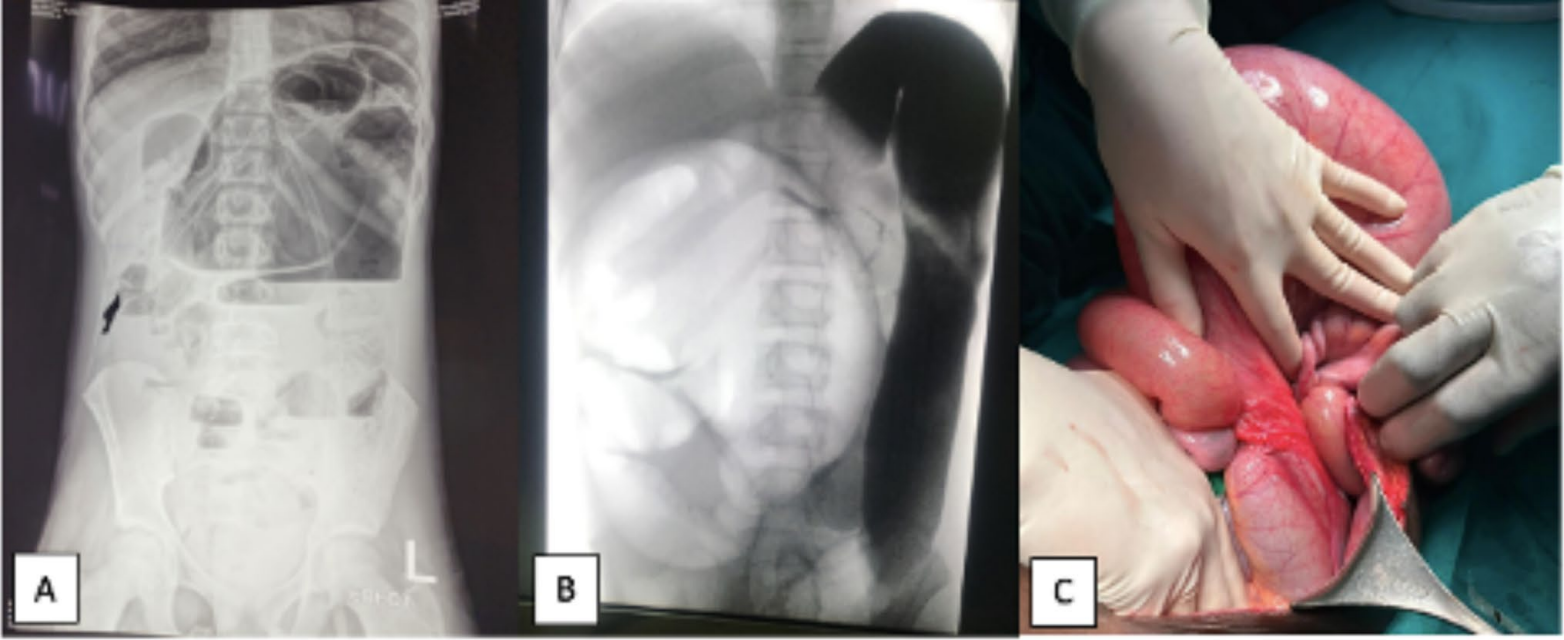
A Abdominal X-ray at presentation: note the dilated loops of the bowel in the left upper quadrant. B Contrast enema: contrastunable to pass the splenic flexure and kidney bean sign in the right lower quadrant. C Intraoperative findings of caecal volvulus
